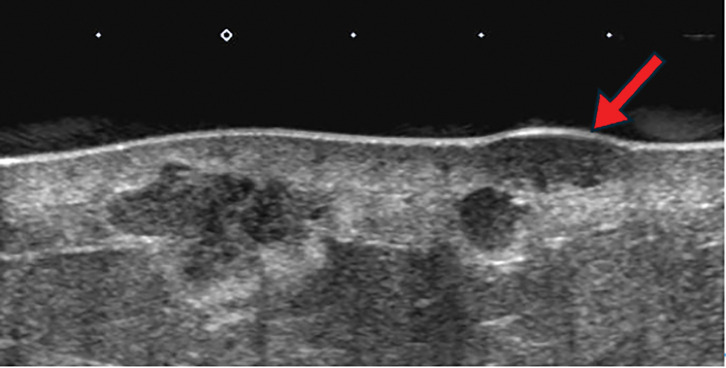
Ultrasonographic findings. Multiple irregular hypoechoic masses, some with suspected skin invasion (red arrow).
